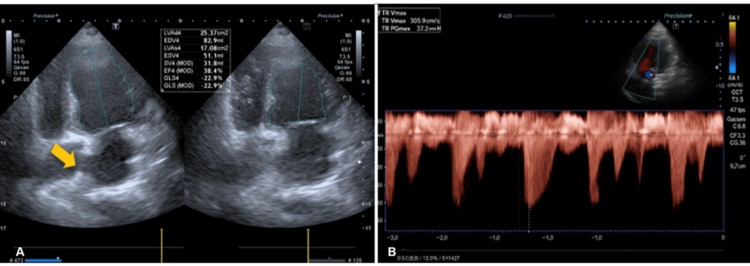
Follow-up transthoracic echocardiogram (A) Apical four-chamber view demonstrating reduced left ventricular systolic function with a left ventricular ejection fraction (LVEF) of 38.4% by Simpson's method. Global longitudinal strain (GLS) is reduced at -22.9%. An interatrial septal defect is visualized (yellow arrow). (B) Continuous-wave Doppler across the tricuspid valve demonstrating elevated tricuspid regurgitation maximum velocity (TR Vmax: 3.05 m/s), corresponding to a peak trans-tricuspid gradient of 37.2 mmHg, consistent with increased right ventricular systolic pressure.
